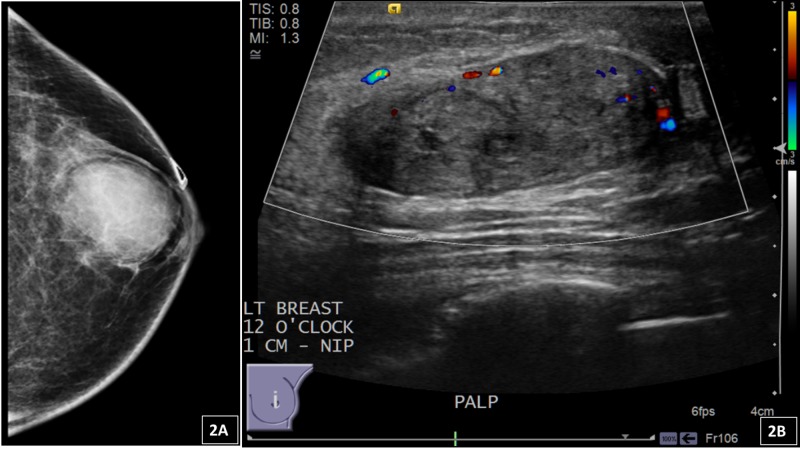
(A) Mammography of the left breast from one of the patients with myofibroblastoma demonstrates an oval mass with circumscribed margins measuring 34 mm. (B) Ultrasound shows an isoechoic oval mass with circumscribed margins and posterior acoustic enhancement. The radiology categorized this lesion as BI-RADS Category 4 B – suspicious for malignancy, biopsy recommended. BI-RADS: Breast Imaging Reporting and Data System
